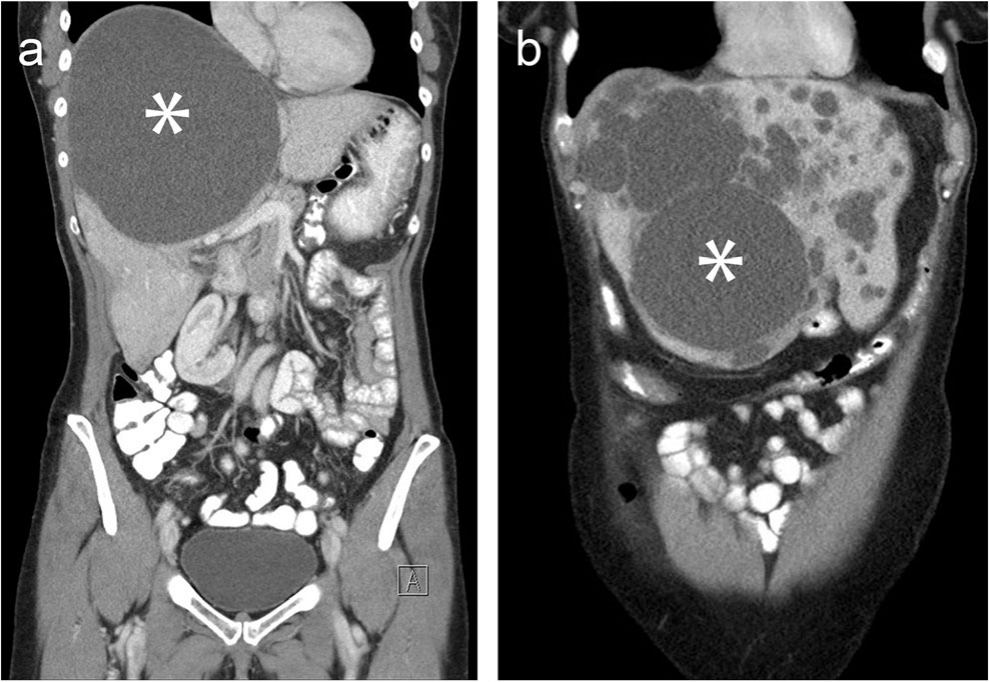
Computed tomography demonstrating (asterisk) a solitary (a) and dominant hepatic cyst in polycystic liver disease (b)
